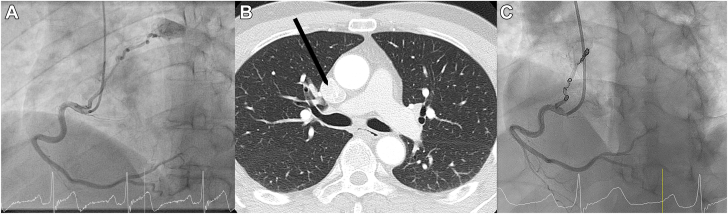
Coronary angiogram and CT scan before coiling and coronary angiogram after coiling of the tumor-feeding artery. (A) Coronary angiogram showing the right coronary artery with a fistula toward a structure near the roof of the left atrium. (B) CT scan showing the cardiac glomus tumor indicated with a black arrow. (C) Coronary angiogram showing the right coronary artery with the tumor-feeding artery after coiling.
